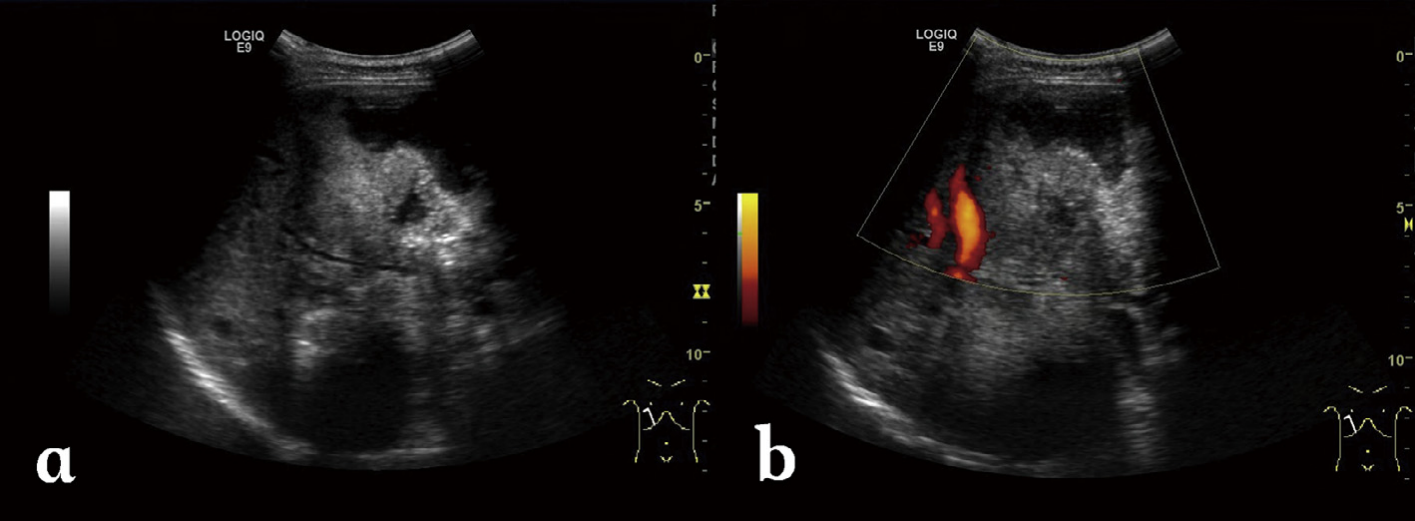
Alveolar echinococcosis in a 30-year-old woman.* (a) Abdominal gray-scale US image shows an irregular type heterogeneous mass lesion with no clear boundary in the right lobe of the liver, containing anechoic pseudo-cystic lesion and hyperechoic foci of calcification. (b) Color Doppler US image shows no obvious blood flow signal while cystic duct is constricted.
